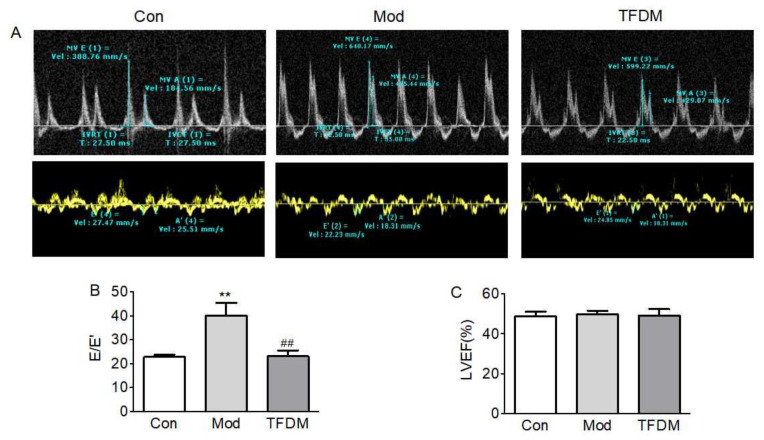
Effects of TFDM on cardiac function. (A) Representative images of pulsed-wave and tissue Doppler recording through the left ventricle. (B) Ratio between mitral E wave and E’ wave (E/E’). (C) Percentage left ventricular ejection fraction (LVEF%) (*n* = 6); compared with Con group:  *p* < 0.01; compared with Mod group ^##^
